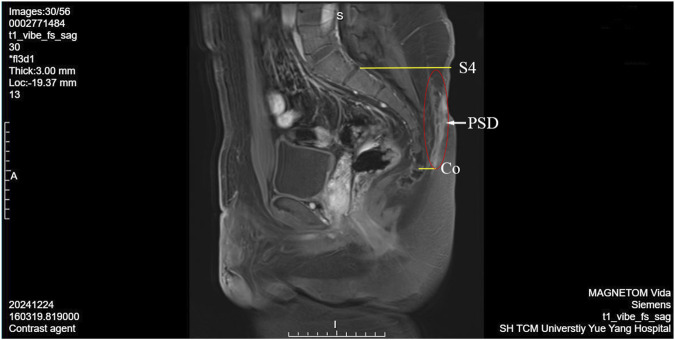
PSD enhanced sacrococcygeal MRI and hydrography image. S4, sacral vertebra 4; Co, coccygeal vertebra; PSD, pilonidal sinuses disease.
